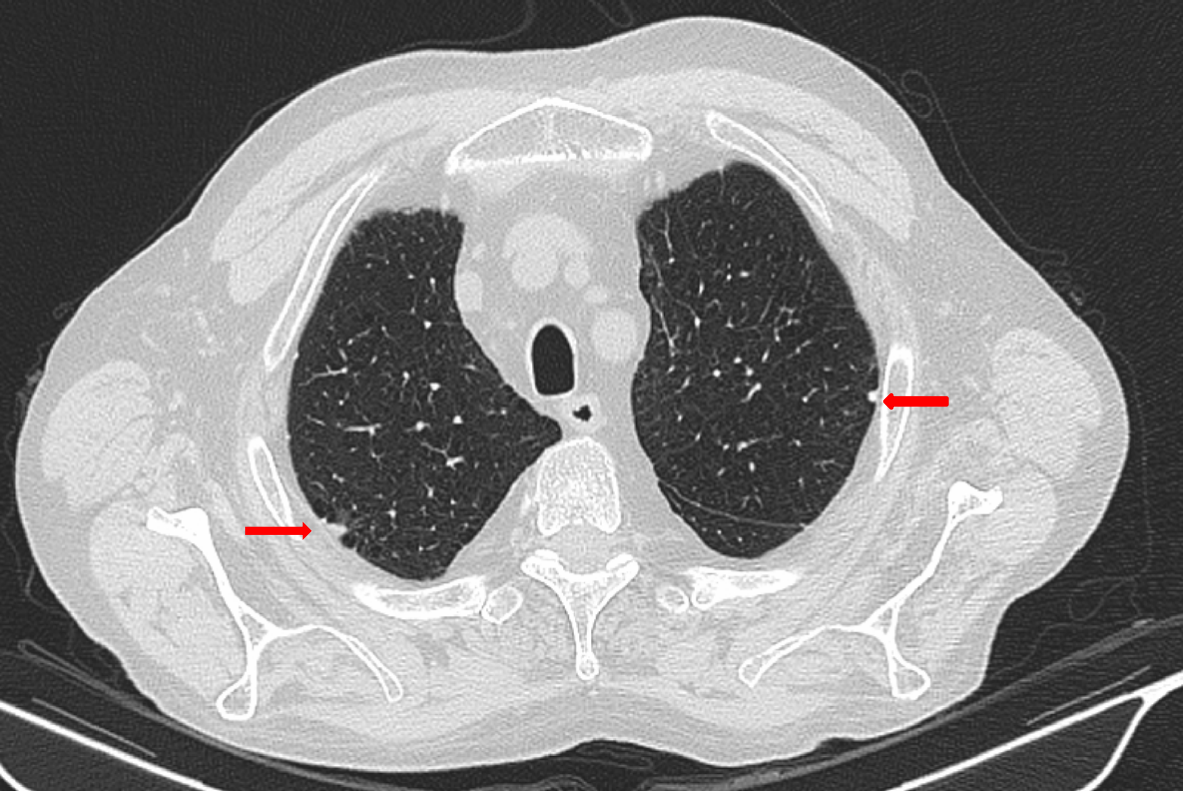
Thoracic HRCT. Presence of pleural plaques
